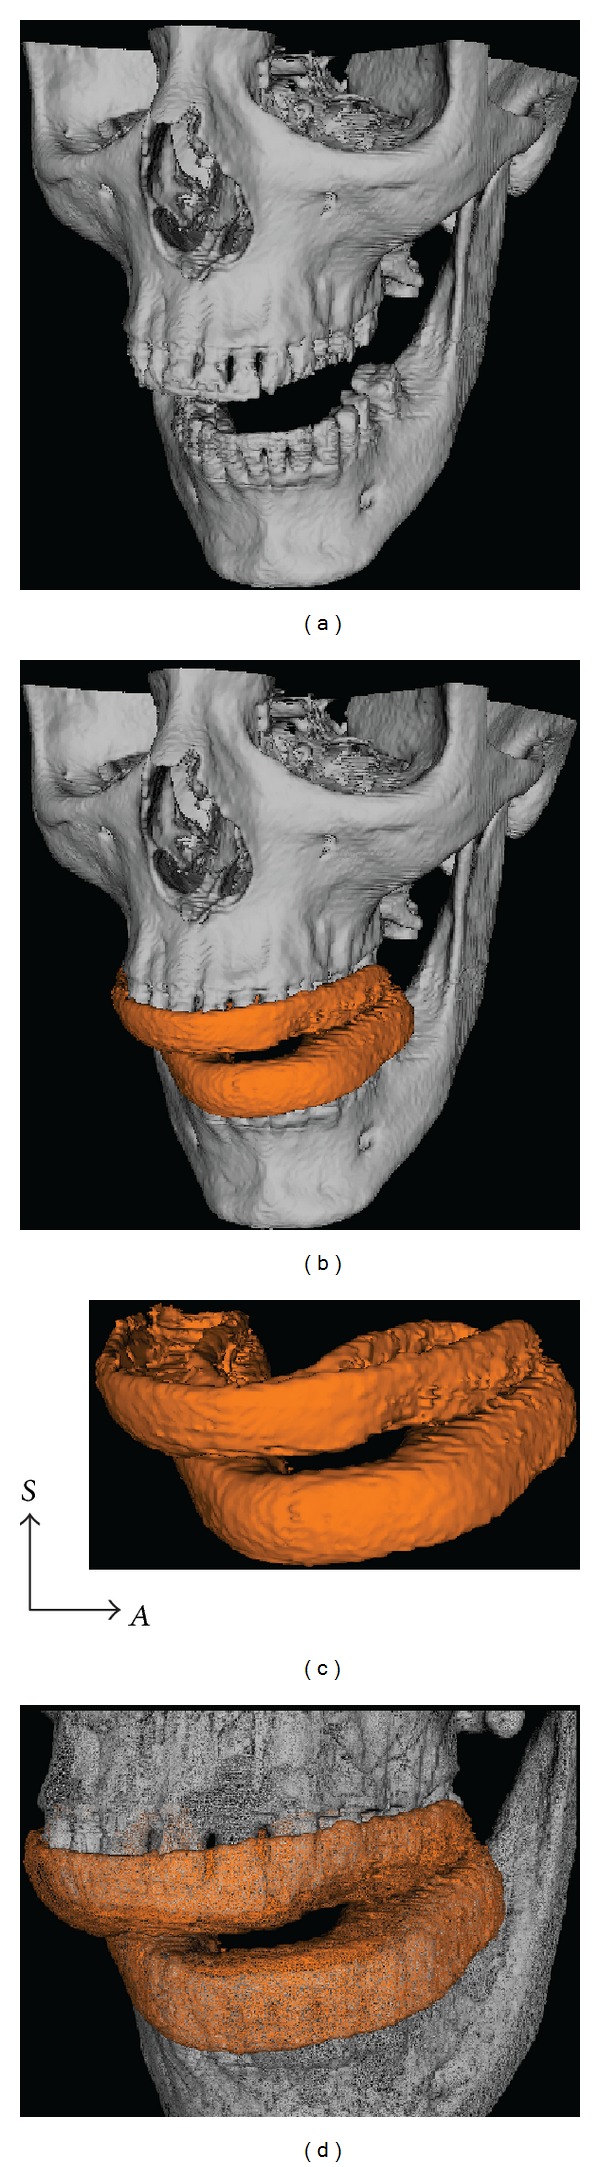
((a)–(d)) The construction of a 3D virtual copy of the provisional guide (orange). (a) 3D reconstruction of the CT images. Notice that the mandible is slighted opened and deflected to the left side. (b) 3D model of the provisional guide (orange) articulated to the dental arches. (c) Provisional guide extracted. (d) Detailed view of the provisional guide adjusted to the upper and lower teeth.
